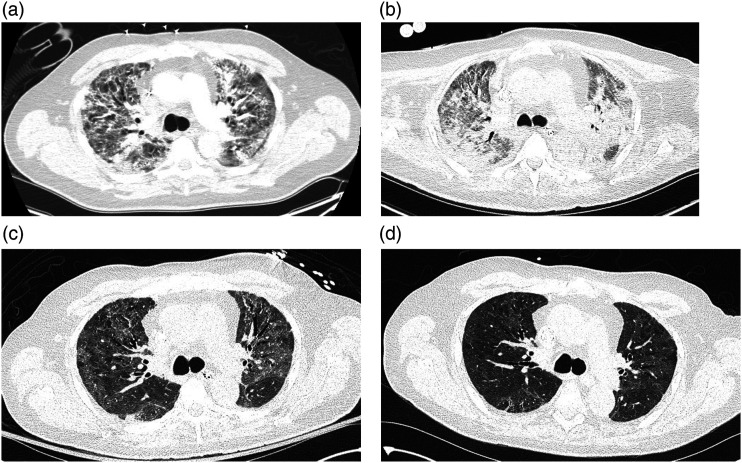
Computed tomography images of the chest at the level of the carina taken
